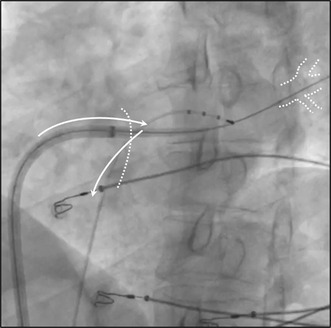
A 55 year‐old male patient diagnosed with symptomatic persistent atrial fibrillation with clinical recurrences during treatment with amiodarone was admitted for scheduled pulmonary vein (PV) isolation using cryoablation. Prior transthoracic echocardiogram showed normal left ventricle systolic function and moderate left atrium (LA) enlargement. CT‐scan showed a normal PV anatomy, and transesophageal echocardiogram showed the absence of thrombus in the LA appendage and no apparent patent foramen ovale. Cryoablation procedure was initiated using the conventional approach. Transseptal puncture was performed with a SL0 sheath and a BRK‐1 needle under fluoroscopy, guided by a wire in the aorta and a tetrapolar catheter in the coronary sinus as landmarks, and using contrast to show the tenting on the fossa ovalis before the puncture. Once it was performed, a guide wire was positioned in the left superior PV. The SL0 sheath was exchanged and the FlexCath Advance™ Steerable Sheath (Medtronic, MN, USA) was progressed over the wire. When we tried to progress the FlexCath into the LA through the wire, we found a high resistance at the atrial septum. Despite several attempts pushing with different torques, the force was insufficiently transmitted and we could not get into de LA with the sheath until we performed the *shoehorn* maneuver (Figure 1). Once this problem was solved, the procedure continued successfully without other incidents.
